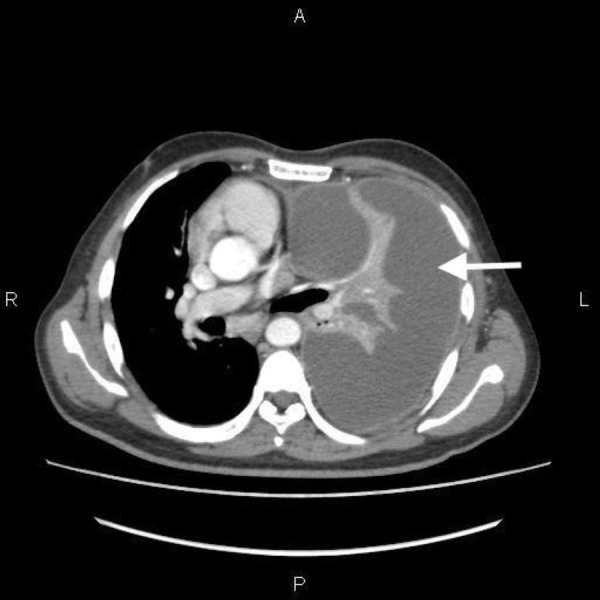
Computed tomography scan of the chest showing a large left-sided multiloculated pleural effusion with collapsed left lung
